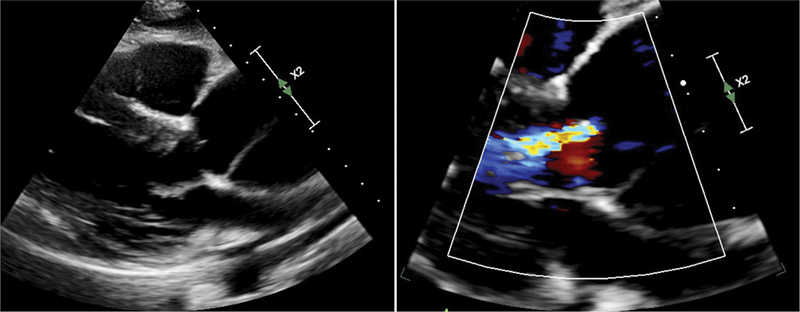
TTE images of Sibling B. Left: PLAX view with severely dilated aortic root (5.8 cm) and visible dissection flap originating at the root and extending into the ascending aorta. Right: Zoomed PLAX view highlighting mild aortic valve regurgitation, a dilated aortic root, and a dissection flap. TTE, transthoracic echocardiogram.
